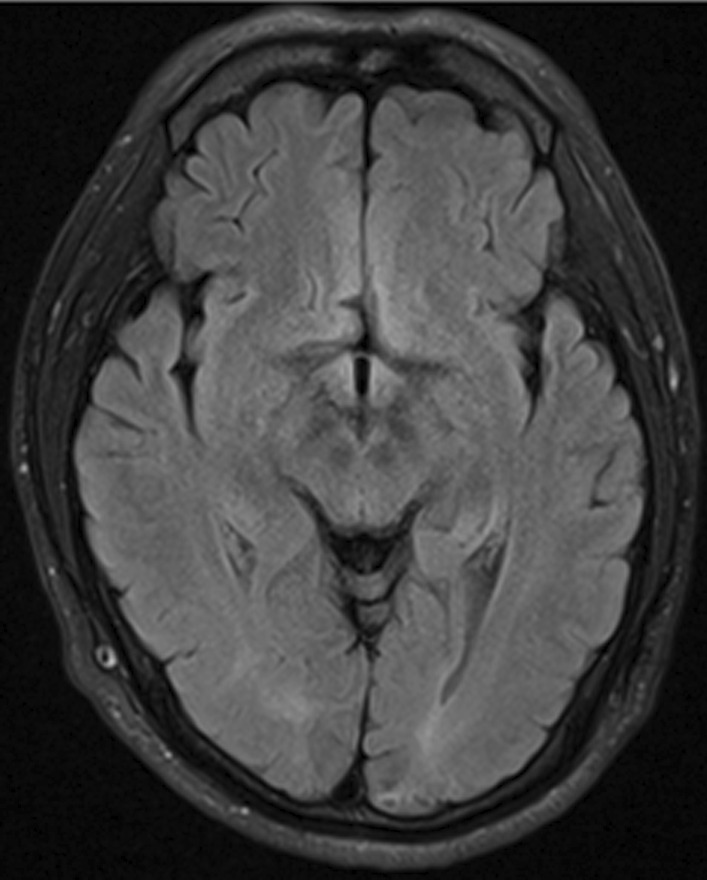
The brain MRI scan after 15 days. No obvious abnormal signal on T2-FLAIR
